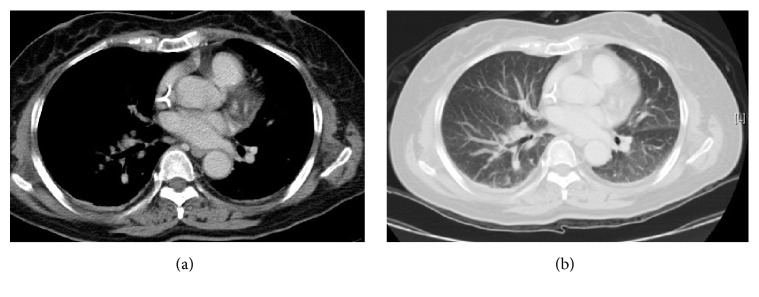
Nodules within pulmonary vein. The patient is a 62-year-old women with lung squamous cell carcinoma who underwent chemotherapy. She felt dyspnea. A chest CT scan (a) and the lung window (b) showed several nodules within her right inferior pulmonary vein.
